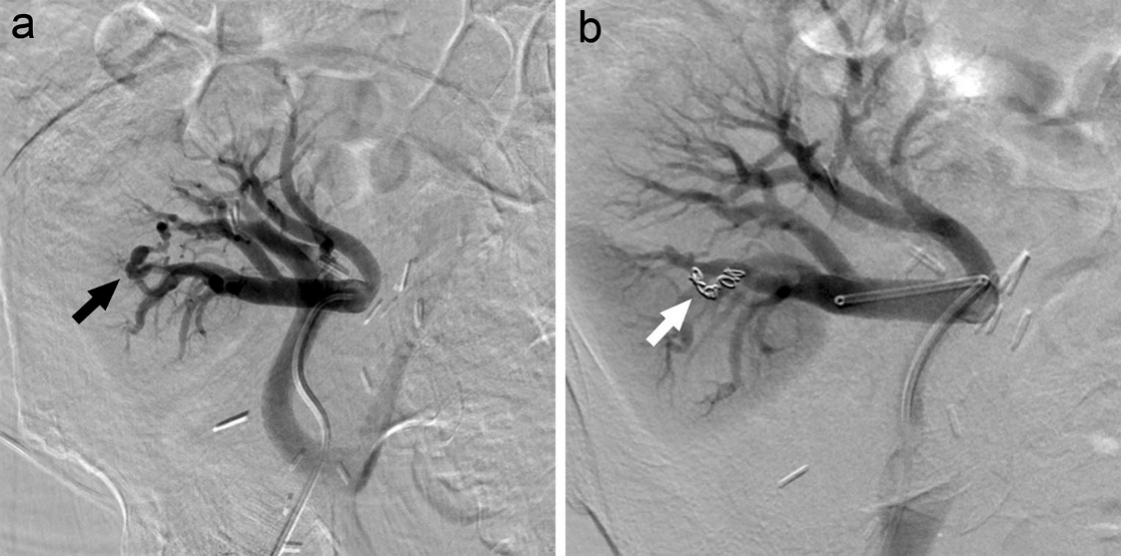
Angiographic image of a right RAVF (a, black arrow) developed following ultrasound-guided biopsy of a transplant kidney with subsequent selective coil embolisation of arterial feeders (b, white arrow)
